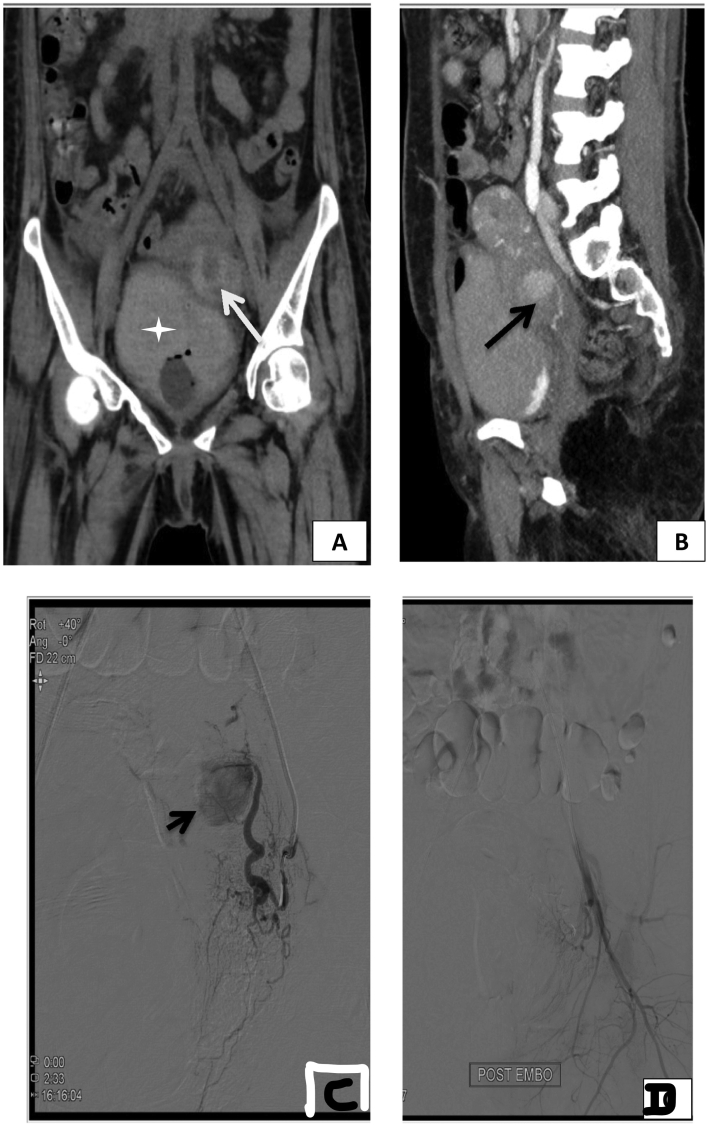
Images of uterine artery pseudoaneurysm. A: Coronal unenhanced CT image showing hyper dense clot in the urinary bladder (white star) and hyper dense haematoma in the pelvis on left side (white arrow). B: Sagittal reformatted maximum intensity projection image of CT angiography, showing a pseudoaneurysm between the bladder and the lower uterus with a tortuous artery reaching up to it (black arrow). C: Digital subtraction angiography image of super selective left uterine artery injection showing the pseudoaneurysm from the distal uterine artery. D: Post-embolization image of left internal iliac artery injection showing non-opacification of the pseudoaneurysm.
